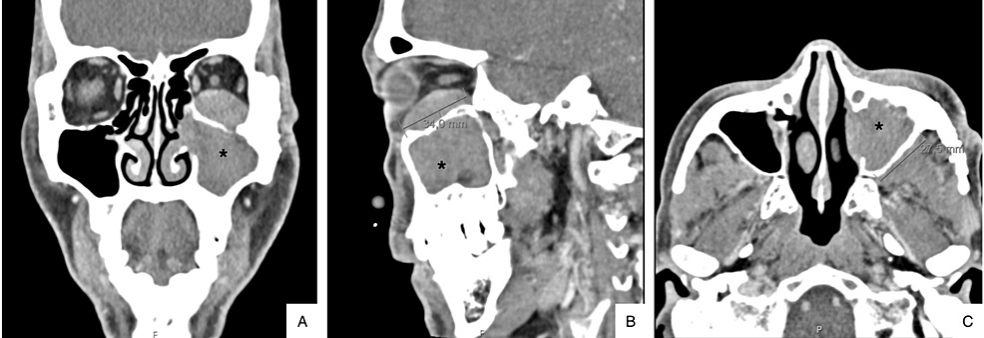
CT scan coronal, sagittal, and axial views showed an extensive soft tissue mass in the left maxillary sinus which extended through the left orbit (asterisk). (A) coronal view, (B) sagittal view, and (C) axial view
